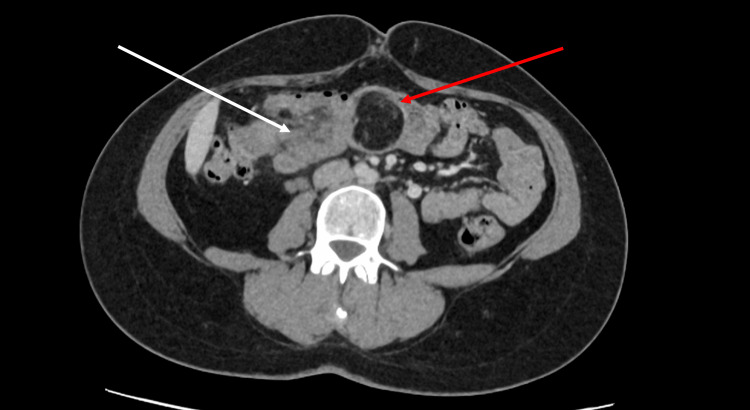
Axial slice CT scan with contrast in portal venous phase, shows colonic intussusception with lipoma as the lead point Red arrow - Shows the appearance of the lipoma, with a darker (hypodense) structure compared to the surrounding tissue. White arrow - Indicates the appearance of intussusception on CT imaging in axial view. You can see the portion of the colon that has slid into the adjacent section of the colon that is enveloping it.
